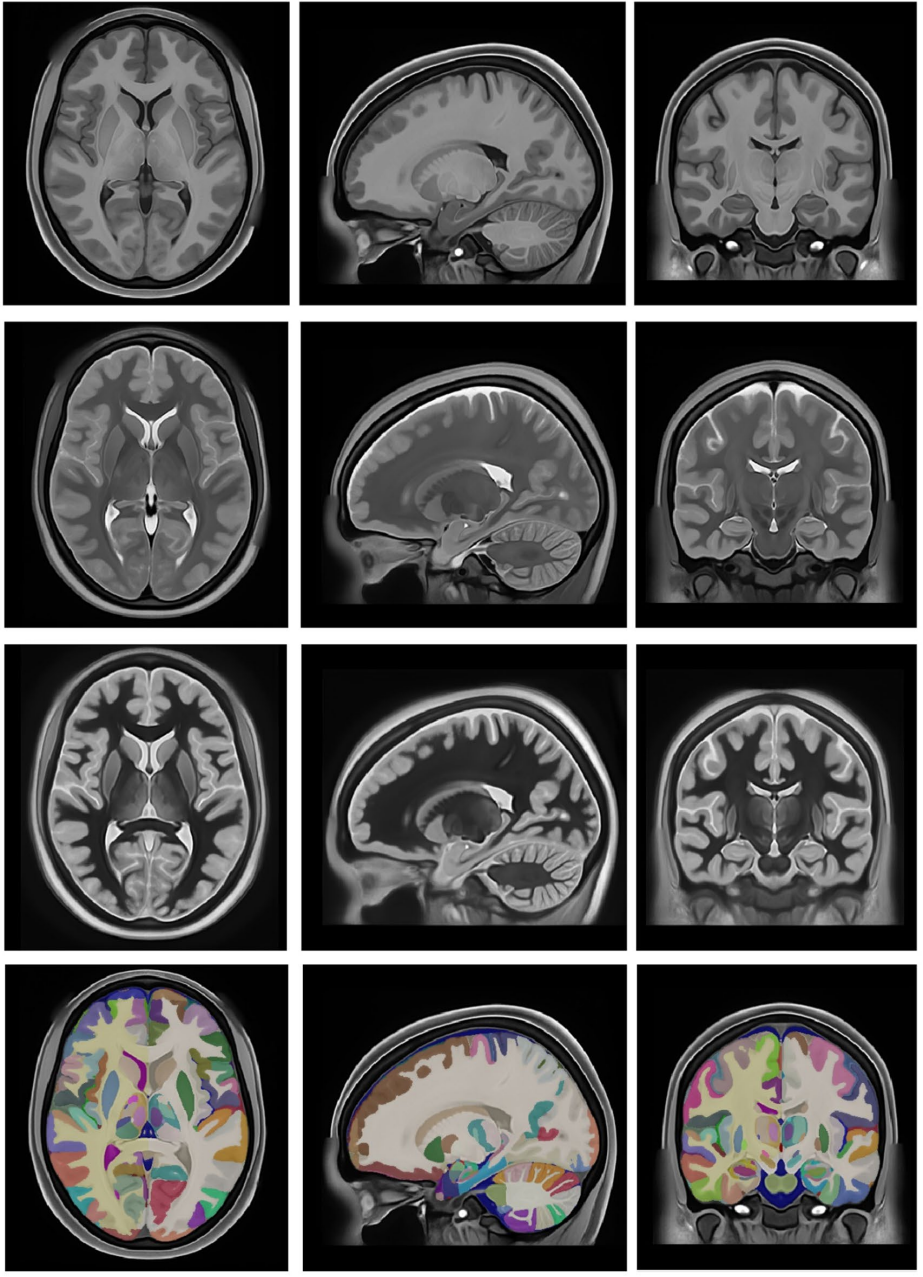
From Top to bottom: Average T1w MRI template, average T2w MRI template, average WMn MRI template and majority voting atlas labels at substructure resolution.
